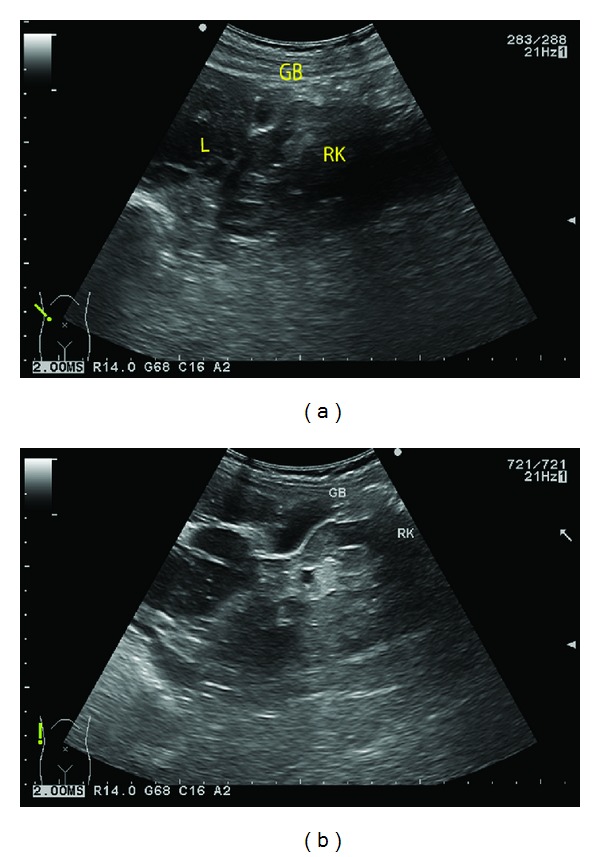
((a) and (b)): ultrasonography with the probe placed at the right lateral abdominal wall revealed a cystic structure (GB) between the upper pole of the right kidney (RK) and the liver (L).
